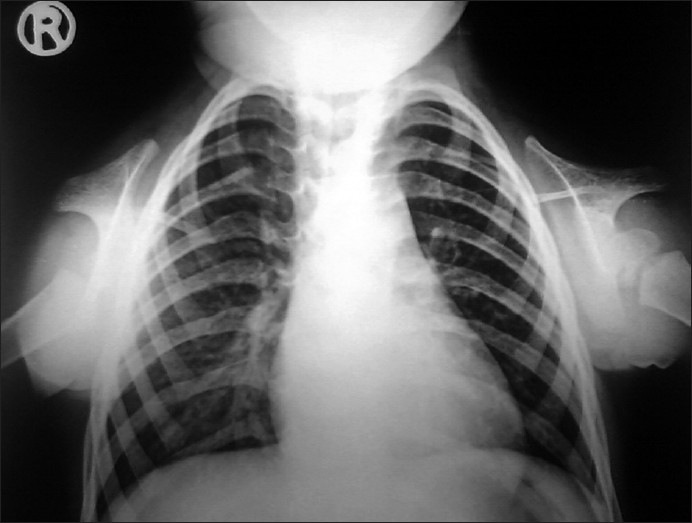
X-ray chest showing bilateral, severely hypoplastic clavicles seen only in lateral aspects
